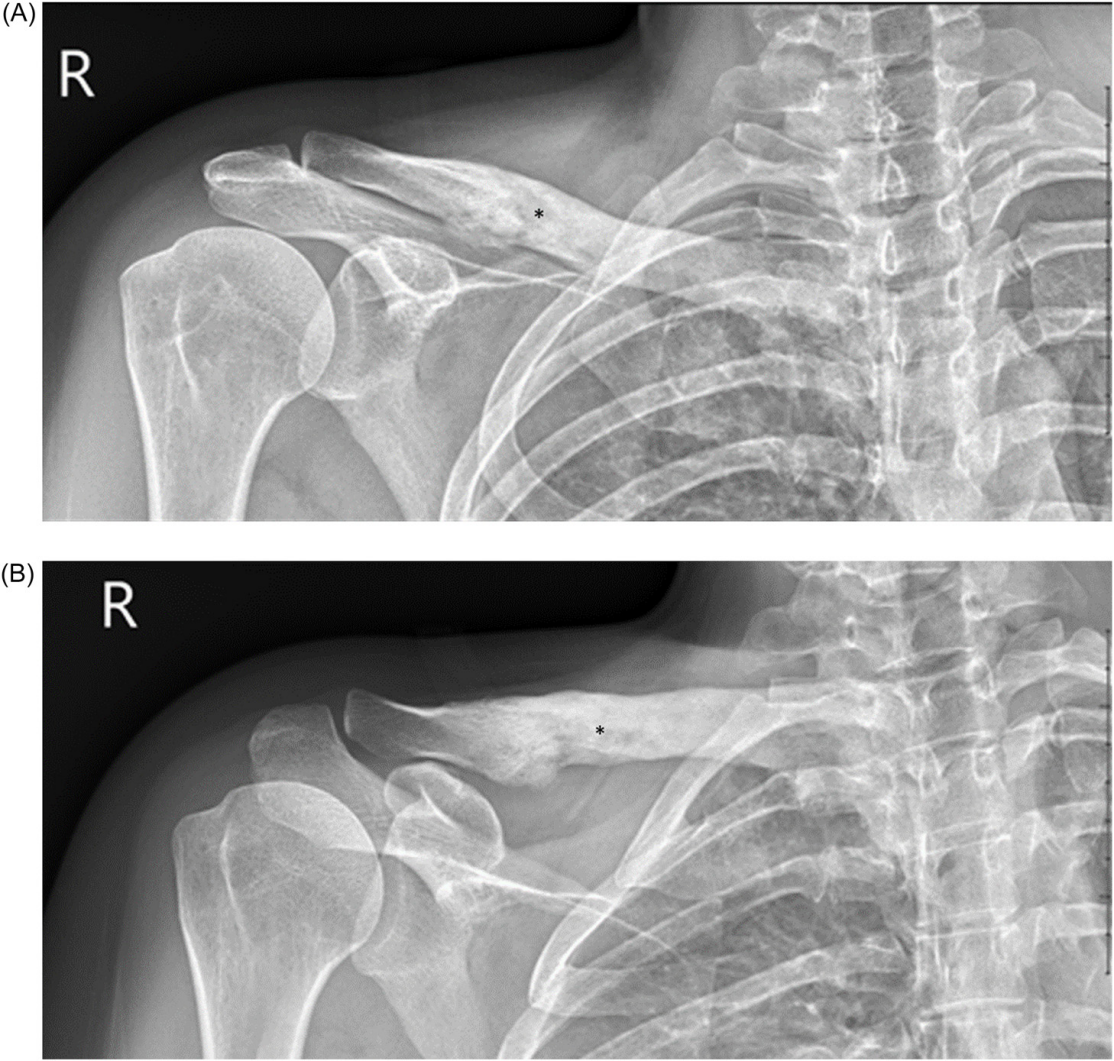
Radiographs of the right clavicle, AP (A) and AP with cephalad angulation (B) views revealing cortical irregularity, erosions and scalloping at the mid clavicle (*).
